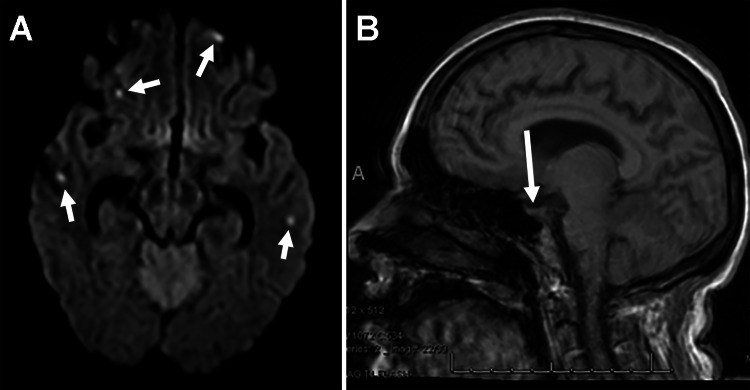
MRI obtained three months after initial diagnosis. (A) Axial diffusion-weighted imaging demonstrating diffuse punctate infarcts from leptomeningeal dissemination around numerous intracranial vessels. (B) Sagittal T1-weighted MRI scan demonstrating a flattened pituitary gland indicative of increased intracranial pressure.
